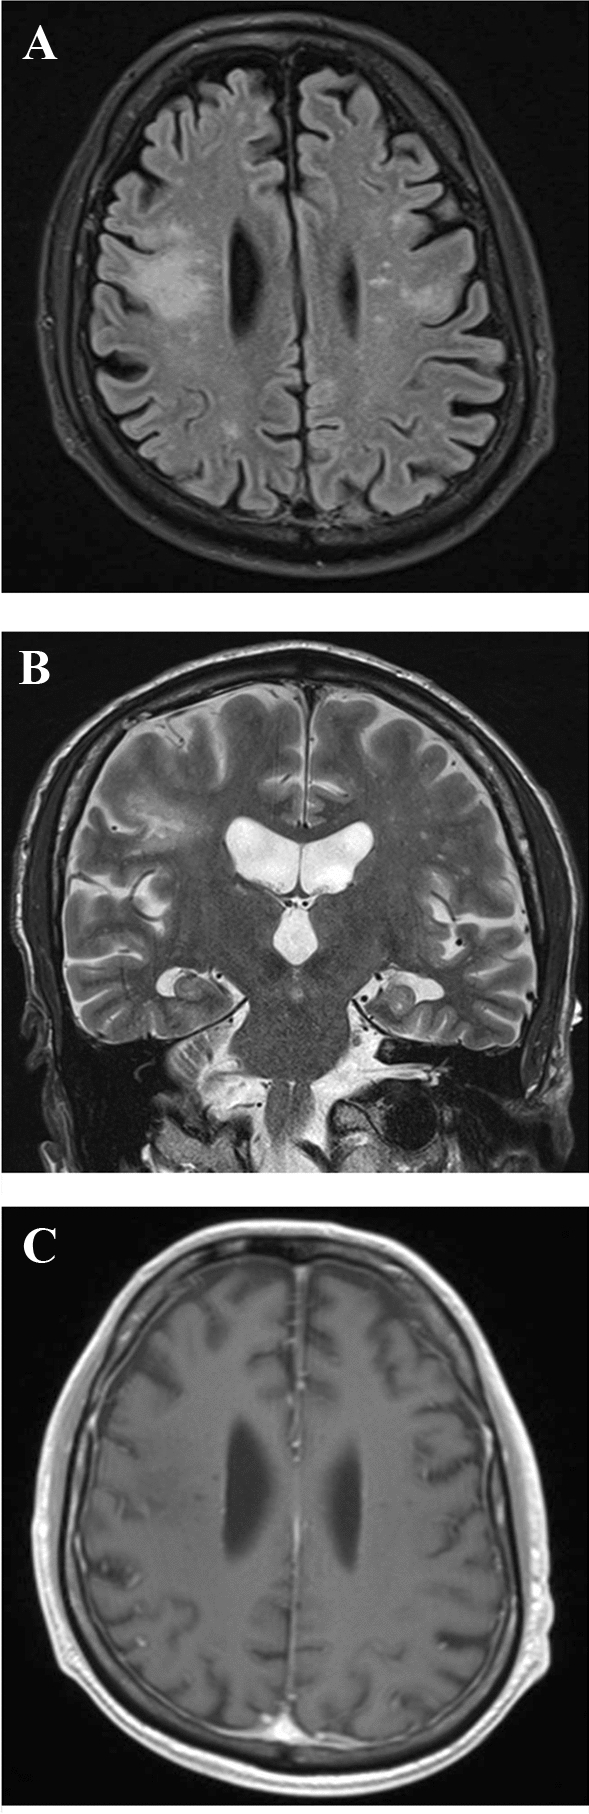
Brain magnetic resonance imaging of the patient. Bilateral hyperintense alterations of the subcortical white matter of the frontal operculum, mostly on the right side, on axial FLAIR (a) and coronal T2-weighted sequences (b). The lesions are hypointense and do not show contrast enhancement on axial T1-weighted sequences (c). The Milky Way sign is evident on both T1- and T2-weighted sequences
